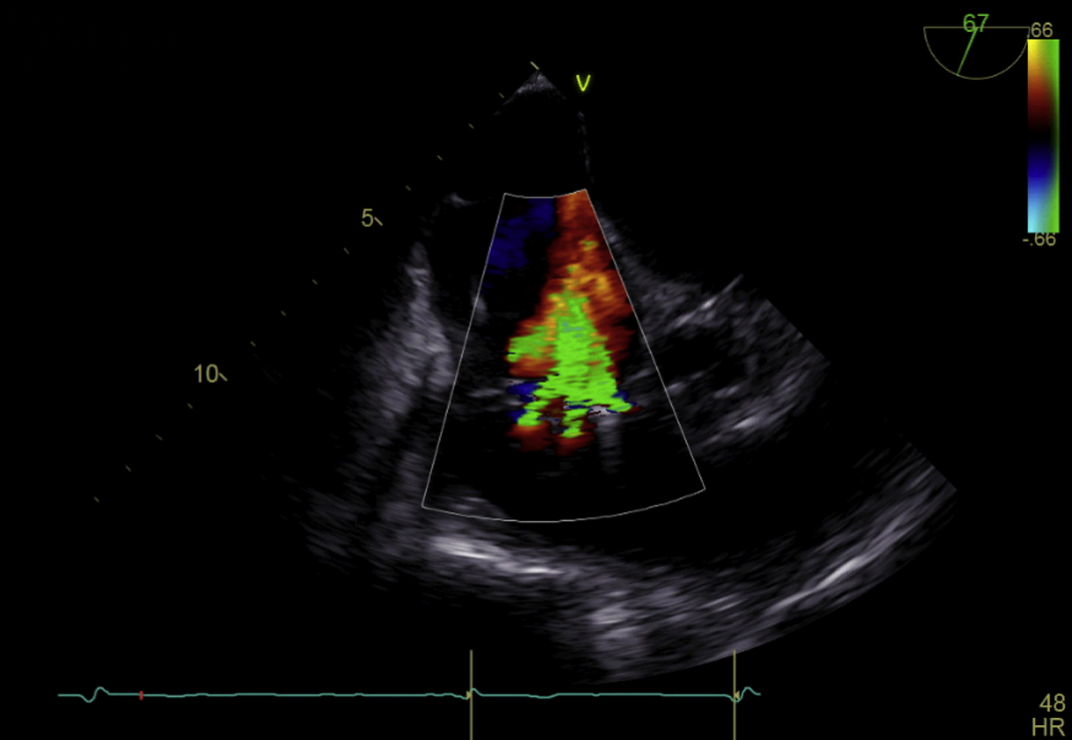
Echocardiography Showing Severe Tricuspid Regurgitation After Single Leaflet Device Attachment
